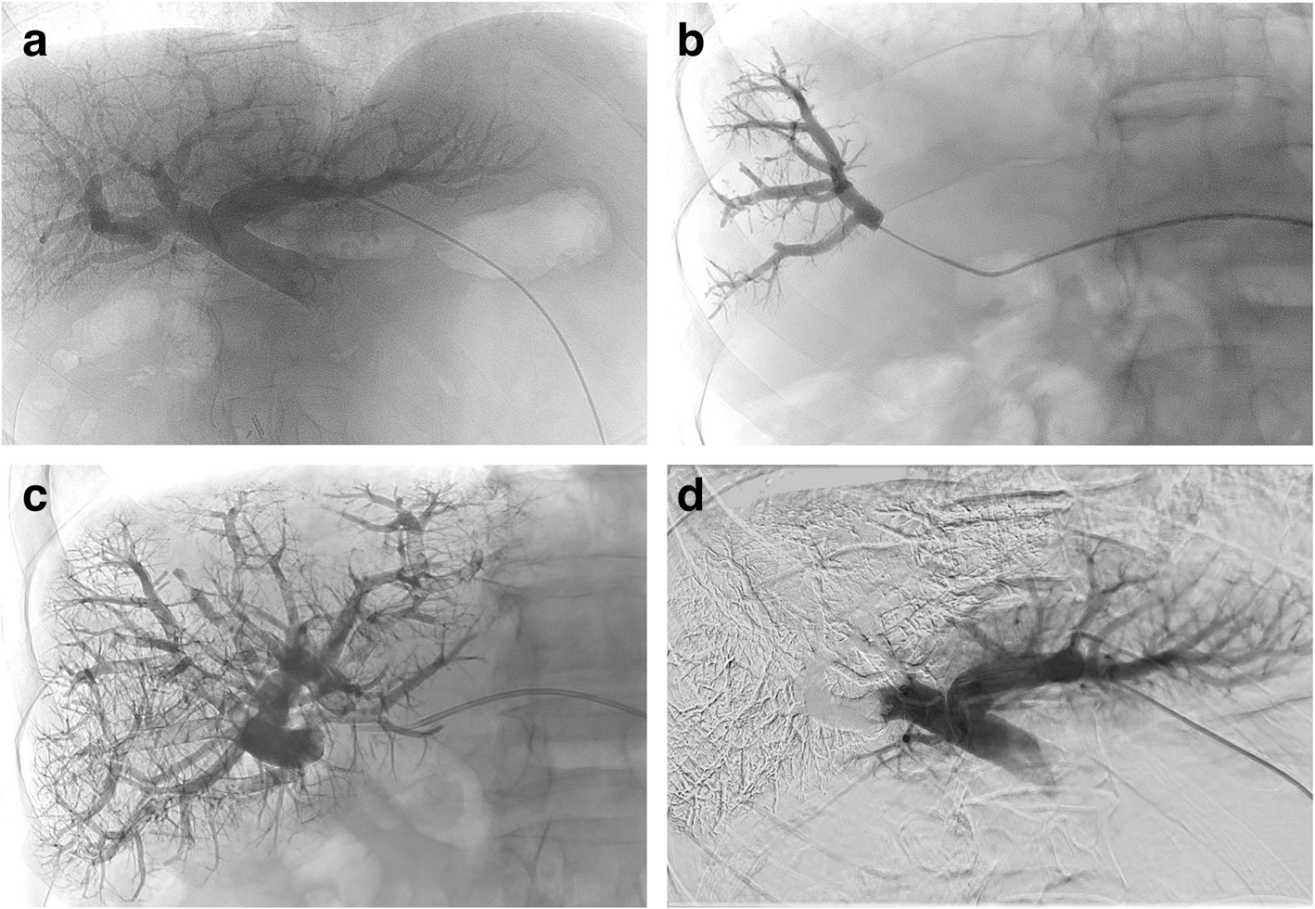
Right portal vein glue embolisation before surgery in a 56-year-old woman with hepatocellular carcinoma. a Portal venography with a 4-Fr standard catheter inserted through a 5-Fr sheath via the contralateral approach: note the normal subsegmental portal branches. b Start of selective glue embolisation of right portal vein branches through a 2.7-Fr microcatheter. c Radiograph after full embolisation of the right portal vein and its branches with AHCA-MagicGlue®/Lipiodol® in a 1:7 ratio showing the distribution of the radiopaque embolisation material. d Final portography revealing complete occlusion of the right portal vein branches and preserved blood flow in the left portal branches and segment IV veins. Hypertrophy of the left liver remnant occurred and right hepatectomy done 6 weeks after embolisation was uneventful
